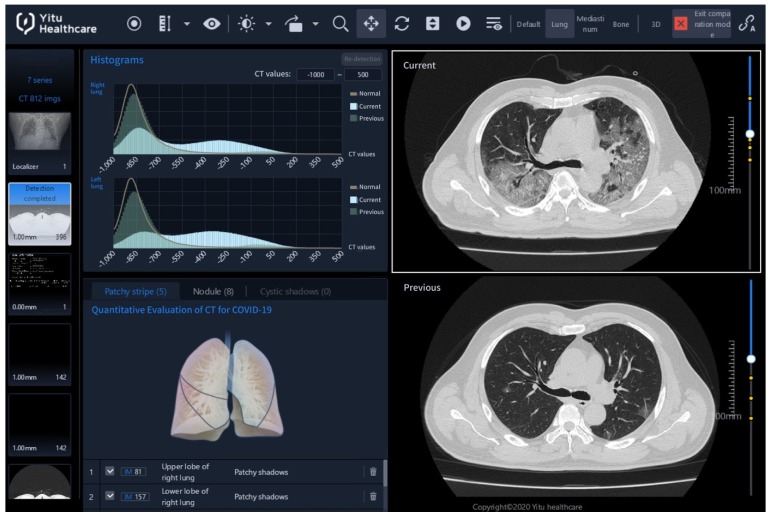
CT image quantization and analysis with artificial intelligence (AI) system. CT images acquired on the day of admission (day 0, in the lower right panel of the figure denoted as “Previous”) and acquired four (±1) days after admission (day 4, upper right denoted as “Current”) can be compared using histograms (upper left) and AI-derived quantitative features. Here, on day 0, the percentage of ground-glass opacity (GGO) volume, percentage of semi-consolidation volume, and percentage of consolidation volume were 0.7, 0.6 and 0.1, while on day 4, they increased to 10.8, 26.1 and 11.5.
